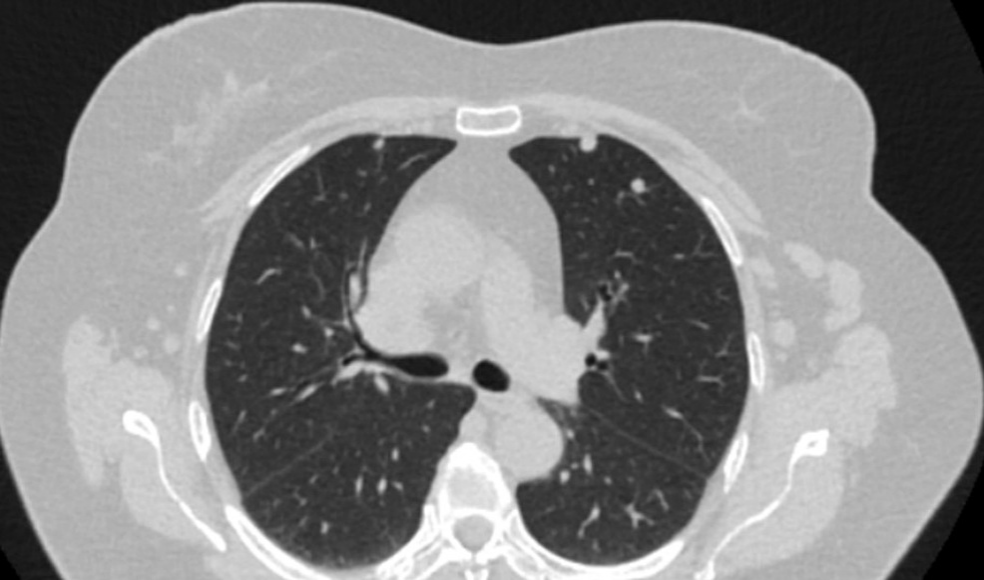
Chest CT scan of the patient with chronic lymphocytic leukemia (nodular lesion is presented)
